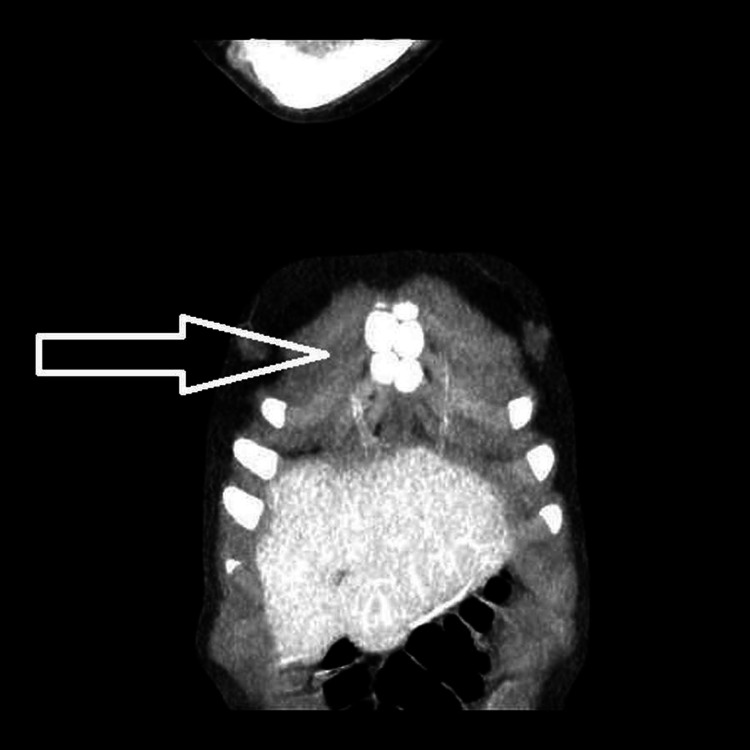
Coronal CT view demonstrating non-fused lateral ossification centers in the sternum CT: computed tomography
